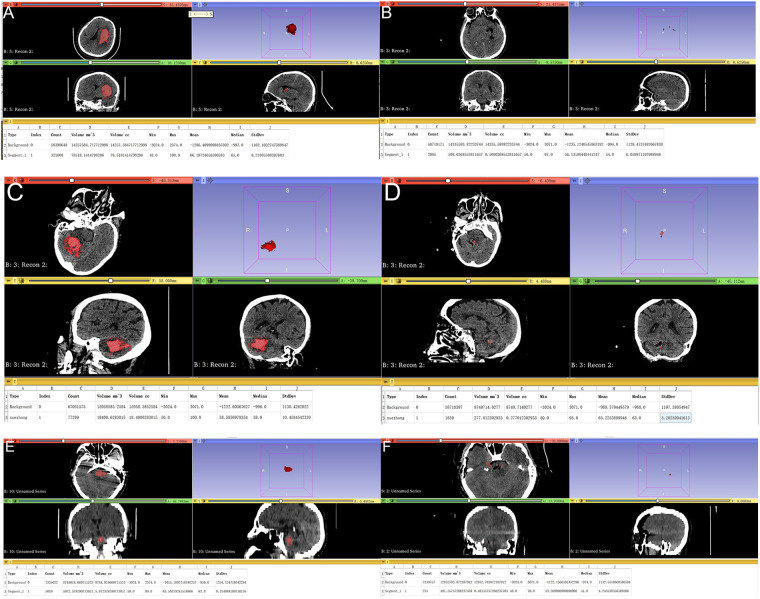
(A,B) Comparative pre- and postoperative CT images of a cerebral hematoma. CT images of a patient with a cerebral hematoma. The preoperative image (A) shows a volume of 78.51 mm^3^, which was successfully reduced to a minimal postoperative volume of 0.50 mm^3^
